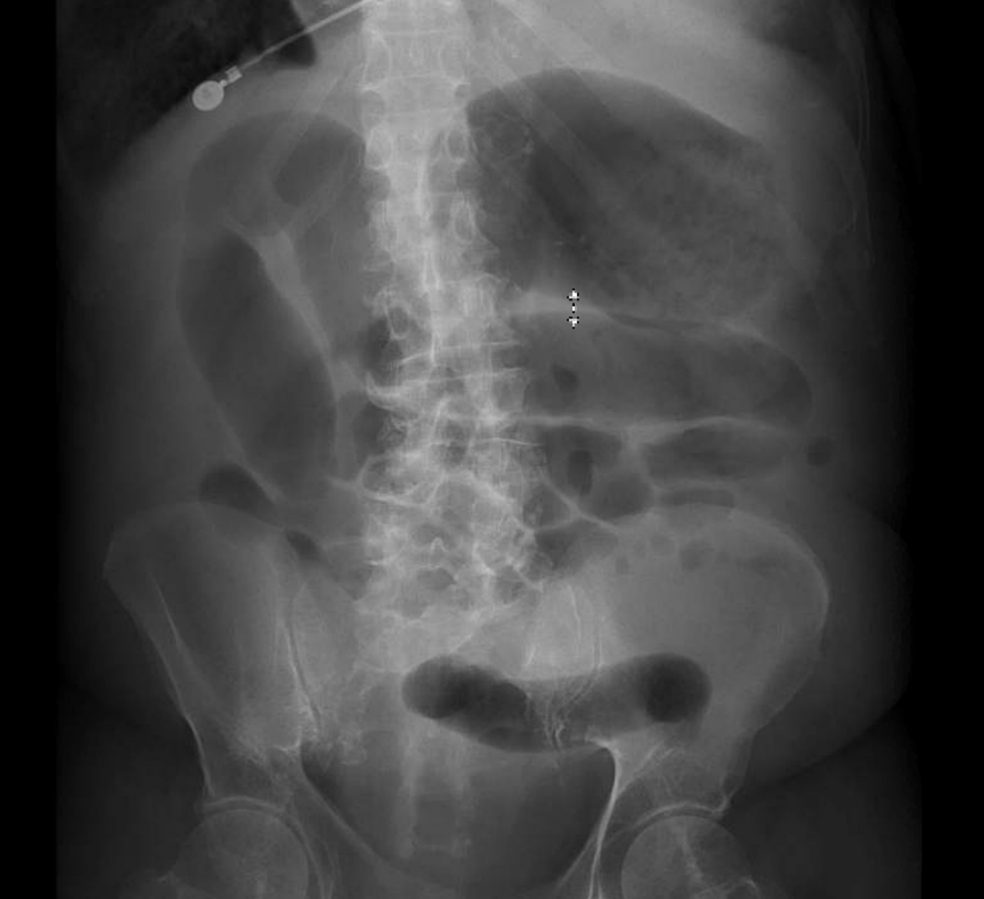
X-ray abdomen showing diffuse intestinal dilatation.
